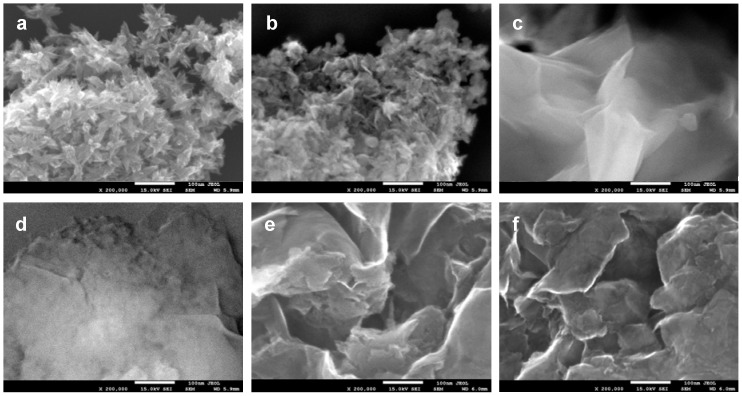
High resolution (HR) SEM images of (a) precipitate obtained from neutralized micellar TiO_2_ precursor; (b) as received MnAl(im); (c) CTA-Mt exfoliated in 1-hexanol; (d) MnAl(im)/Ti-IMEC_NaOH_-II-600; (e) MnAl(im)/Ti-IMEC_NH3_-II-600; (f) MnAl(im)/CTA-Mt-600.
